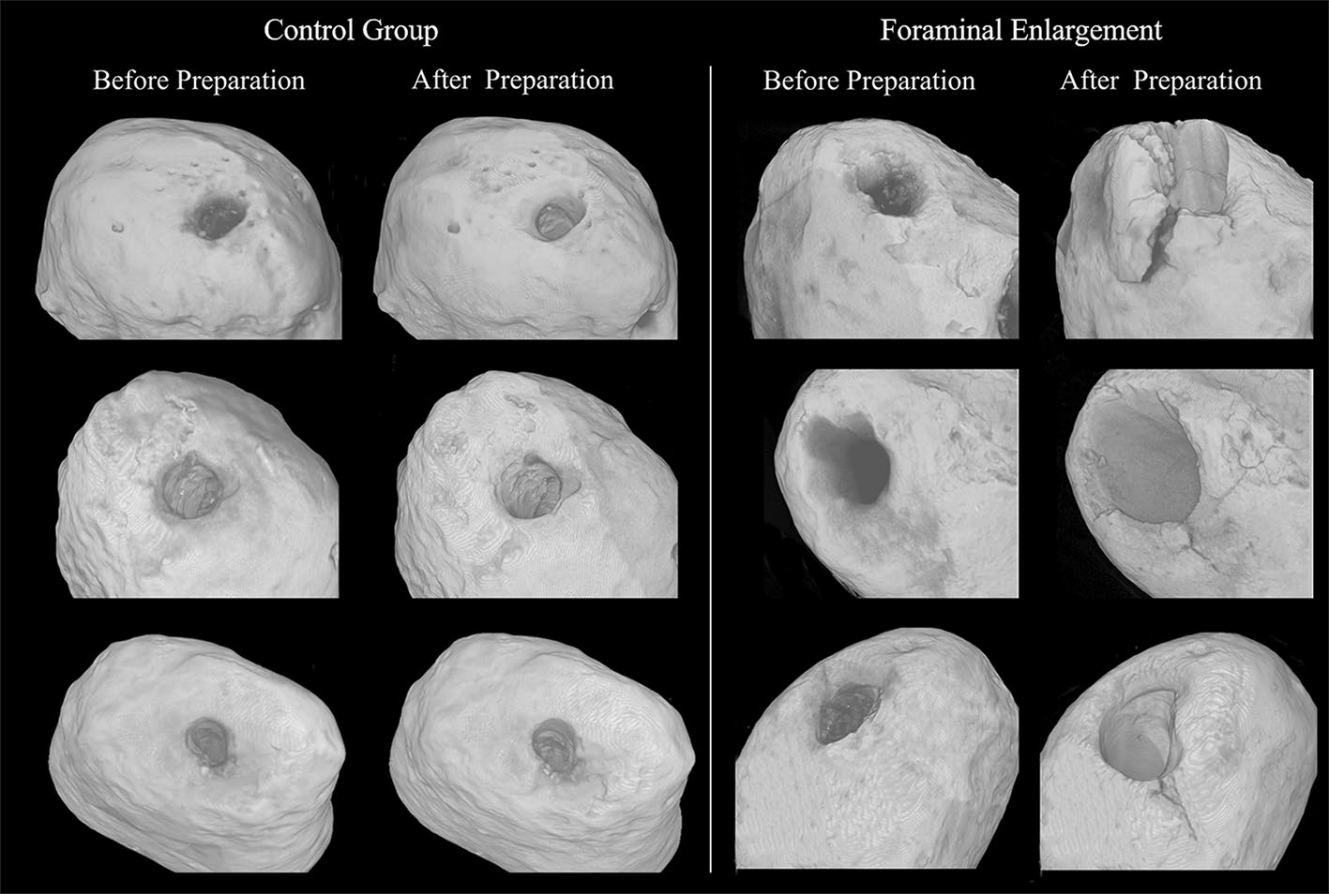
3D reconstruction of nano-CT showing the presence of microcracks and apical deformations after foraminal enlargement.
